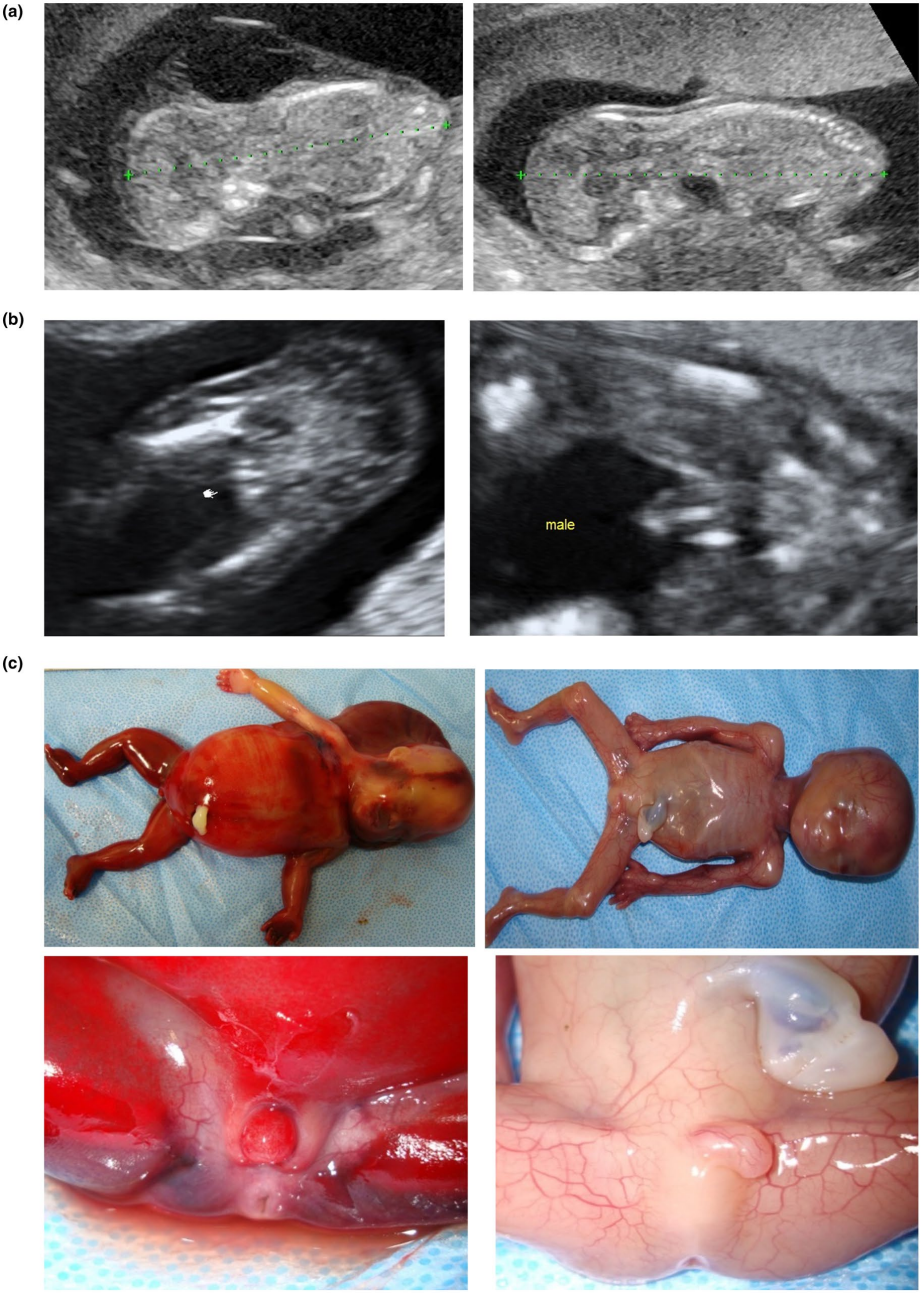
(a) Ultrasonography images revealing cystic hygroma in fetus 1 (left) and healthy fetus 2 (right). (b) Ultrasonography images of fetuses with different external genitalia. External genitalia of female fetus 1 (left) and male fetus 2 (right). (c) In fetus 1 (left), severe cystic hygroma and clitoral hypertrophy were detected. In fetus 2 (right), major anomalies could not be detected without bilateral cryptorchidism
